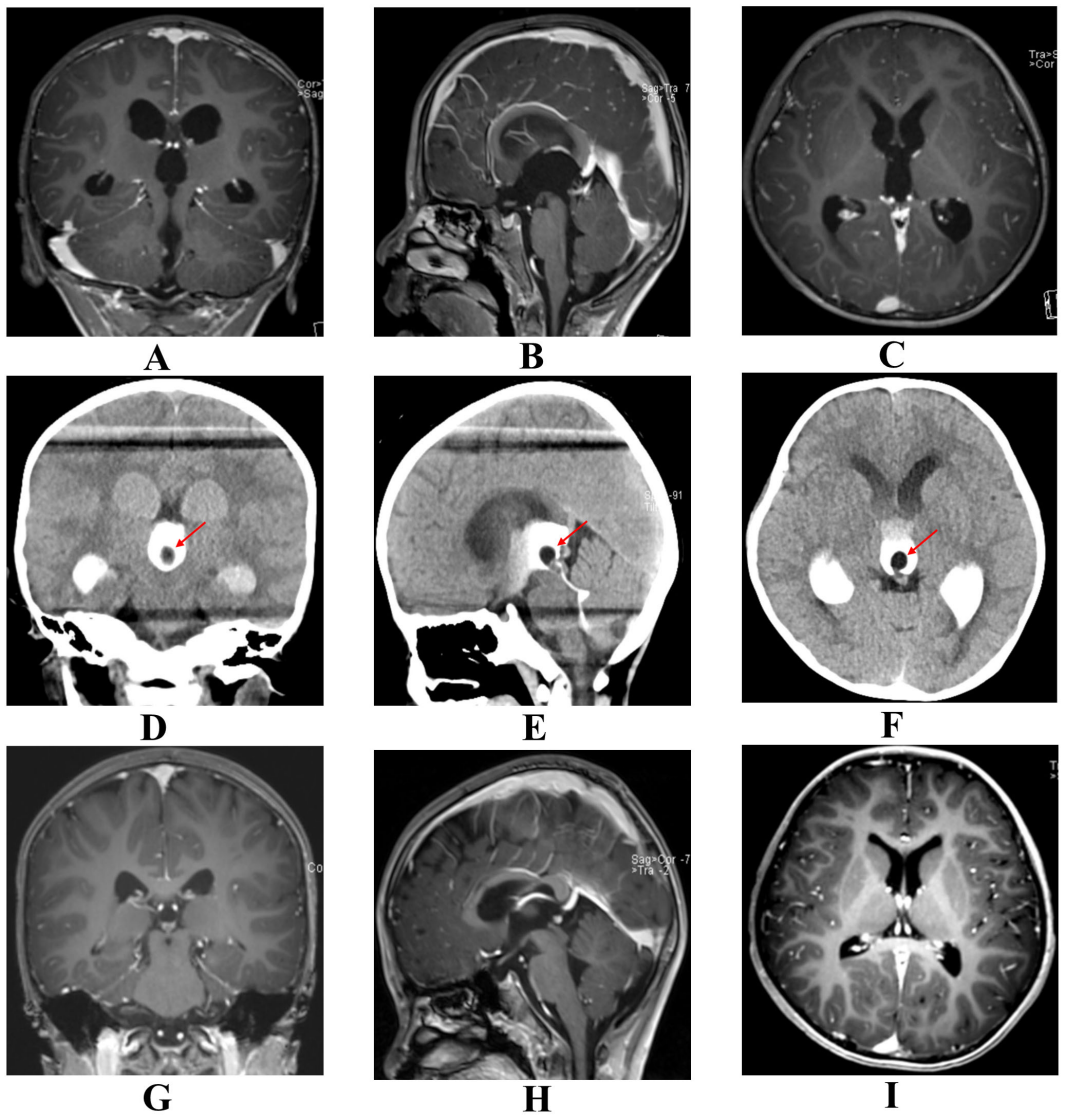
Pre- and postoperative radiological comparisons of Case 2. Images are aligned in consistent anatomical planes (Left column: Coronal; Middle column: Sagittal; Right column: Axial) to facilitate direct comparison. (A–C) Preoperative MRI showing supratentorial hydrocephalus with a funnel-shaped aqueduct but no definitive mass. (D–F) Preoperative CT ventriculography revealing a 0.8-cm filling defect at the aqueductal inlet (arrows), which identified the occult obstruction. (G–I) Postoperative contrast-enhanced MRI at 72 hours showing a significant reduction in ventricular size and patent CSF pathways, confirming successful treatment.
